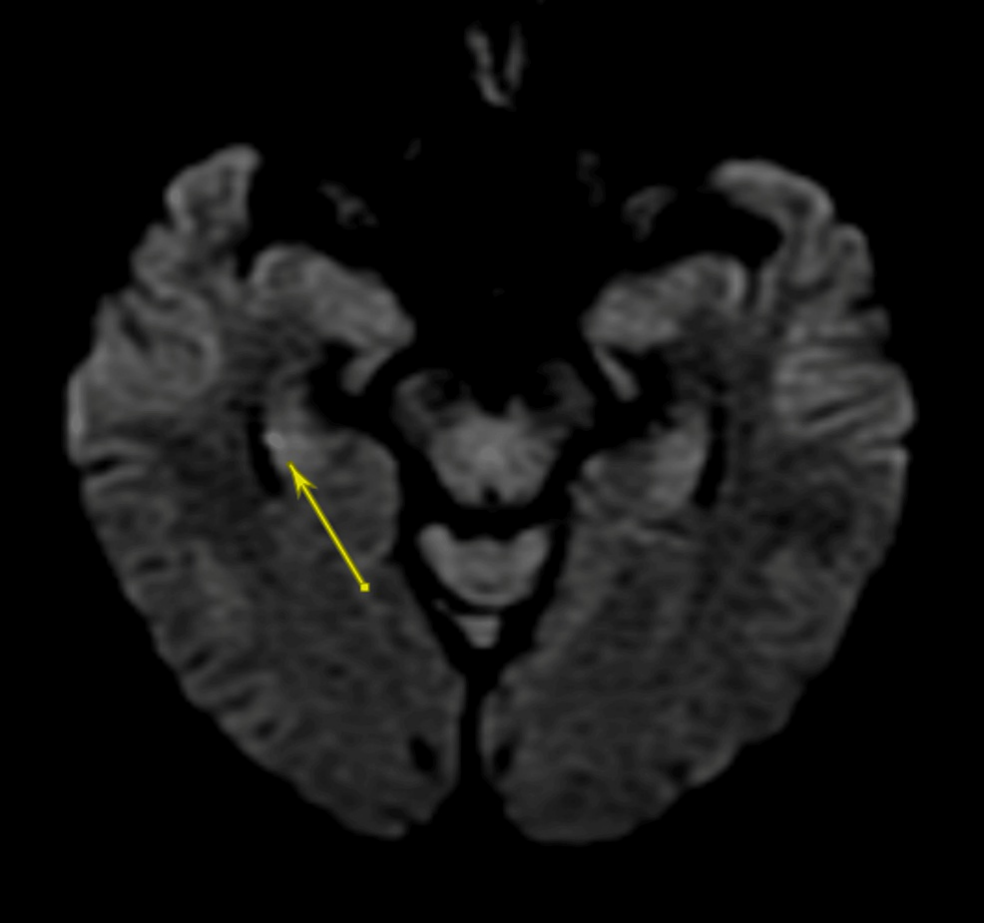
Axial view magnetic resonance diffusion-weighted imaging (DWI) showing a right 2 mm acute infarct of the midportion of the hippocampus
